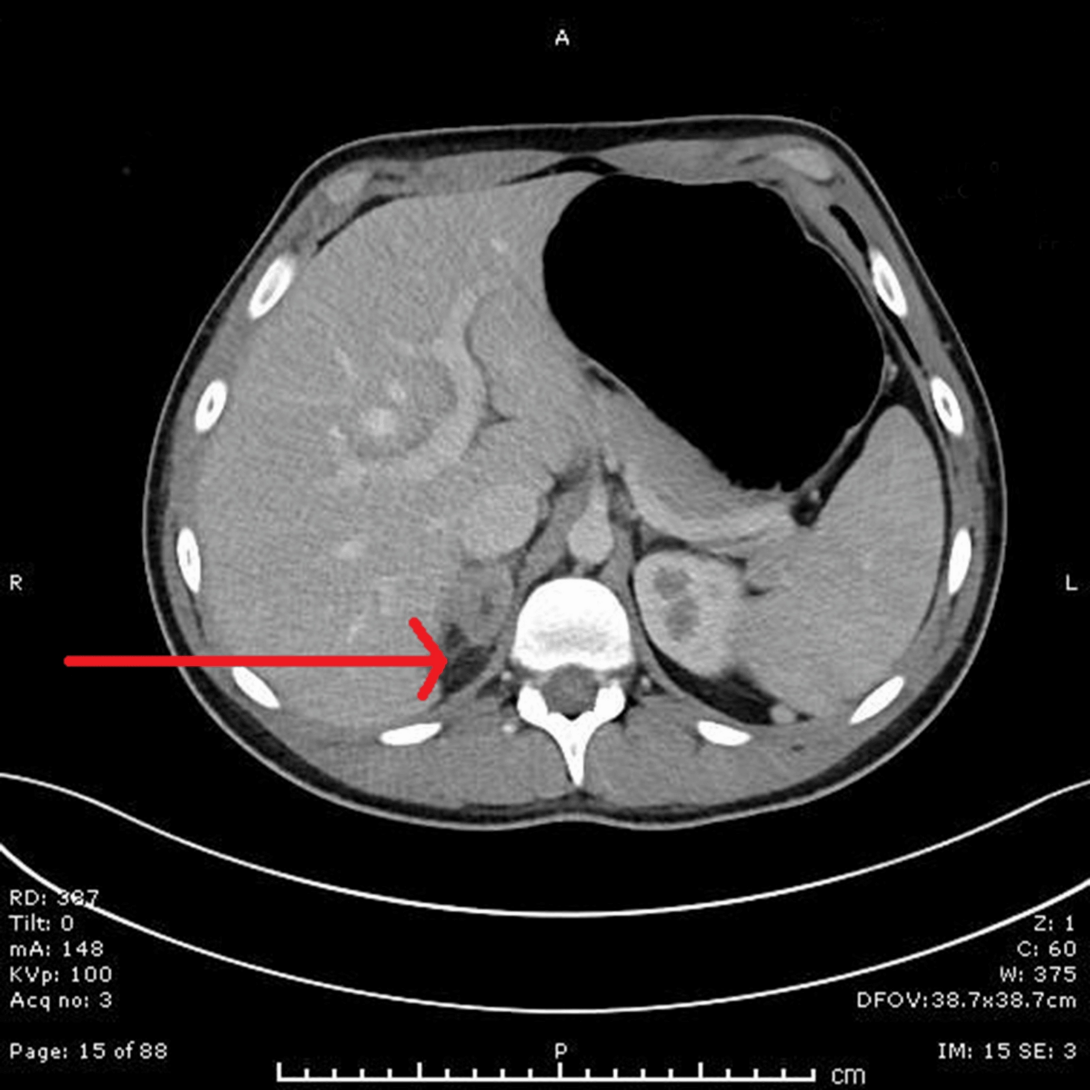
Contrast-enhanced CT (day 60): Further reduction in right adrenal abscess volume; left adrenal remains clear
